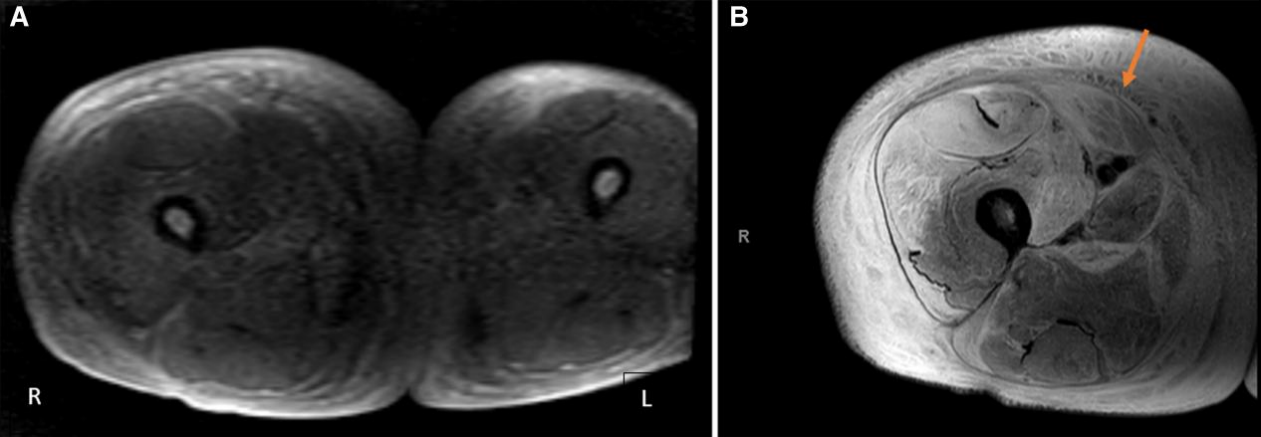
Magnetic resonance imaging survey image showing architectural distortion of anterior fibers of the rectus anterior femoris muscle.
